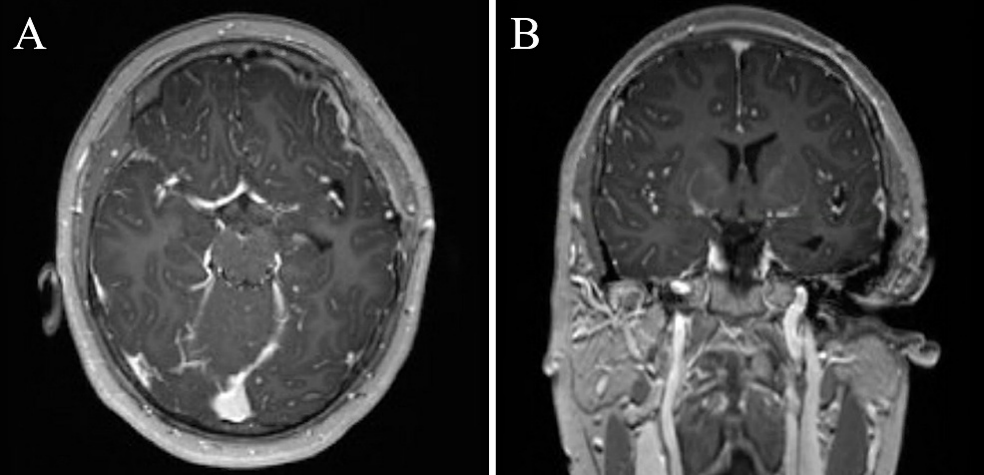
Three-month follow-up T1-weighted MRI axial (A) and coronal (B) views without MA-M recurrence MA-M: meningiomas combined with meningioangiomatosis
